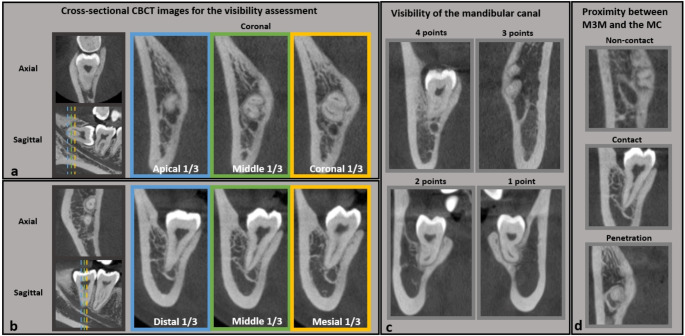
Cross-sectional CBCT images determined for the mandibular third molar (M3M) on each registered scan for image assessment (a and b). For tilted or horizontally impacted M3Ms, the coronal slices were determined at the apical, middle, and coronal thirds of the M3M root(s) (a). For vertically impacted M3Ms, the coronal image slices were determined at the distal, middle, and mesial aspects of the M3M, respectively (b). Representative CBCT images displaying varying visibility of the mandibular canal (c) and M3M-MC proximity (d)
